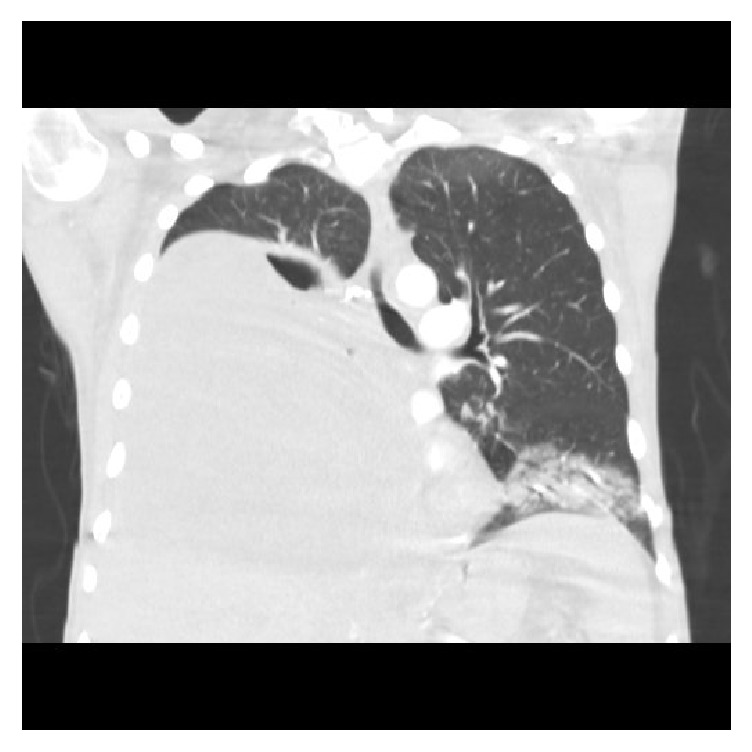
CT on admission showing a large right sided pleural effusion containing pockets of gas along with focal airspace disease within the lingula and left lower lobe.
